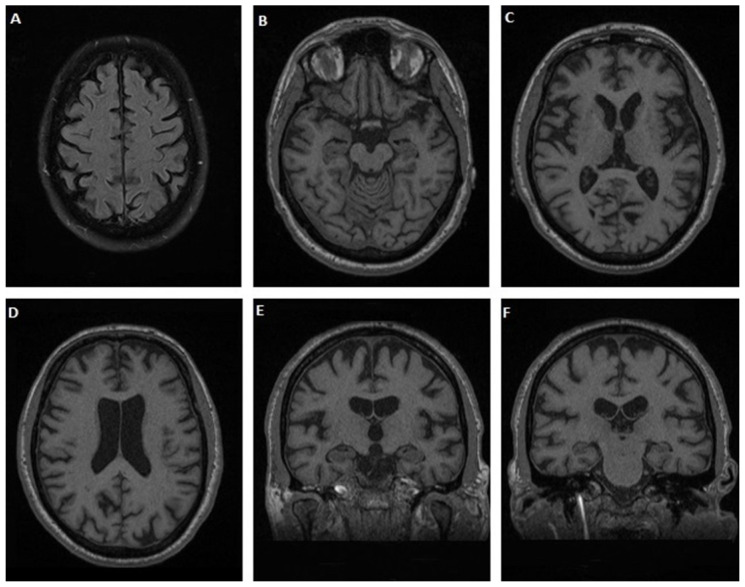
Brain MRI of case 6. Axial (A–D) and coronal (E,F) T1 images showing significant cortical atrophy in frontal, parietal and temporal lobes.
